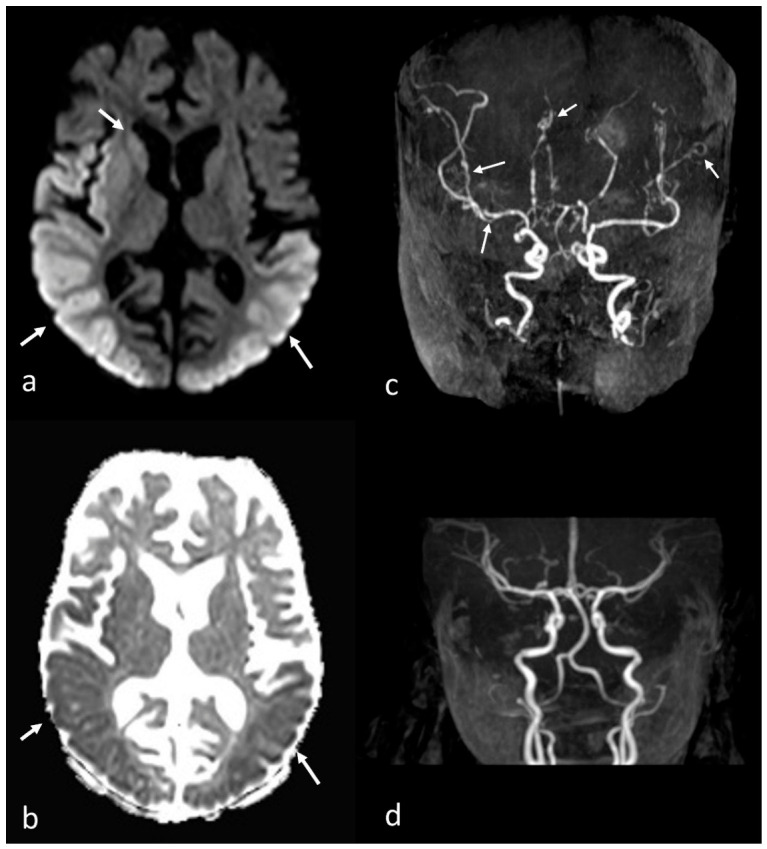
Cranial magnetic resonance imaging and angiography findings at 12 months of age. Following hiatal hernia repair, the patient developed postoperative neurological deterioration. Axial diffusion-weighted imaging (a) and apparent diffusion coefficient map (b) demonstrate diffusion restriction involving the bilateral cerebral cortex and right striatum, compatible with hypoxic–ischemic encephalopathy. Time-of-flight magnetic resonance angiography with maximum intensity projection reconstruction shows cerebral vessel tortuosity and distal branch narrowing in the middle cerebral artery, anterior cerebral artery, and posterior cerebral artery territories (c). Magnetic resonance angiography of an age-matched healthy child is provided for comparison and demonstrates normal vascular morphology (d).
